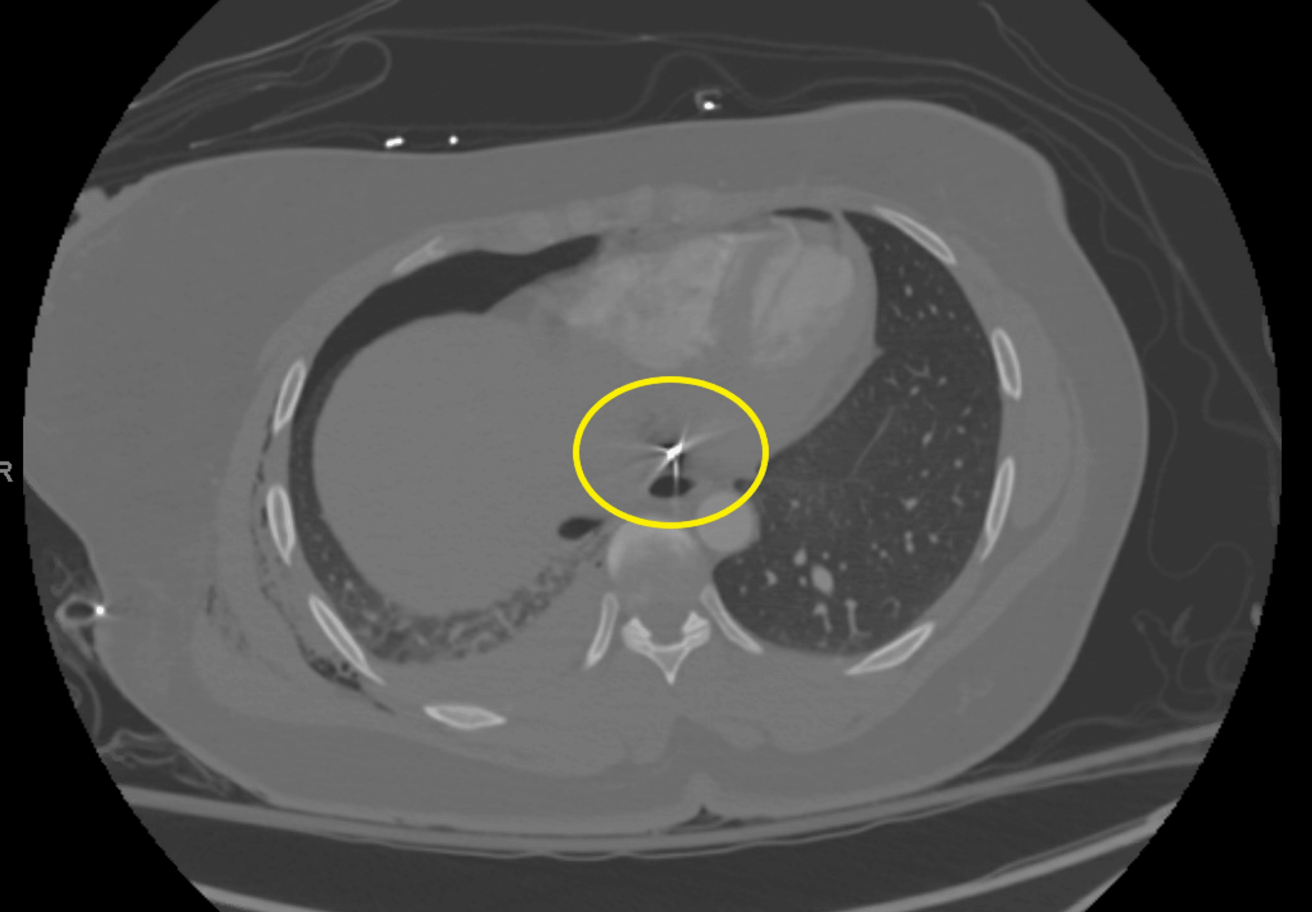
Preoperative CTA An additional hyperdense object was located in proximity to the inferior vena cava.
